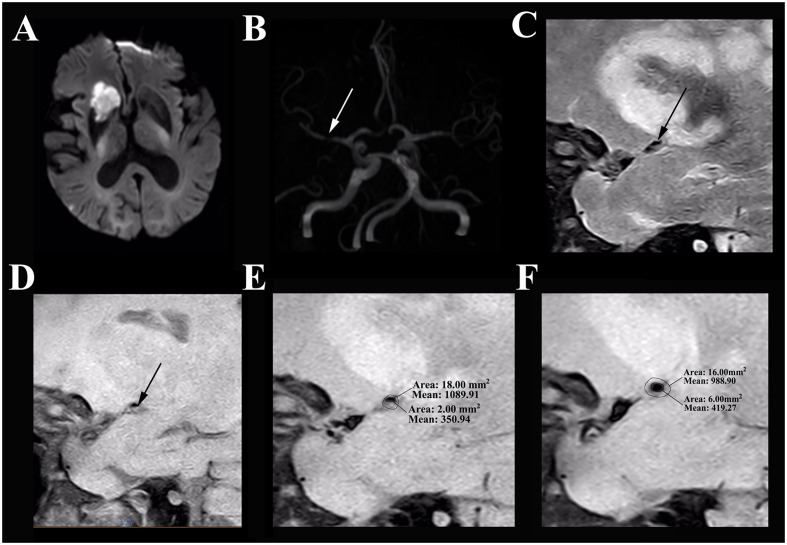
HR-MRI of a symptomatic MCA stenosis in an 85-year-old male who presented with left limb weakness for 10 hours. (A) DWI shows an acute ischemic stroke in the distribution of the right MCA. (B) The TOF-MRA manifests severe stenosis of the M1 segment of right MCA (white arrow). (C) The plaque in T2-weighted HR-MRI (black arrow). (D) The plaque in T1-weighted HR-MRI (black arrow). (E). Measurement at the most narrowed site in proton density-weighted HR-MRI: the vessel area is 18.00 mm^2^ and the lumen area is 2.00 mm^2^. (F) Measurement at the reference site in proton density-weighted HR-MRI: the vessel area is 16.00 mm^2^ and the lumen area is 6.00 mm^2^. The remodeling index = 18.00 mm^2^/16.00 mm^2^= 1.13 (positive remodeling); the degree of stenosis = (1–2.00 mm^2^/6.00 mm^2^) = 66.67%.
